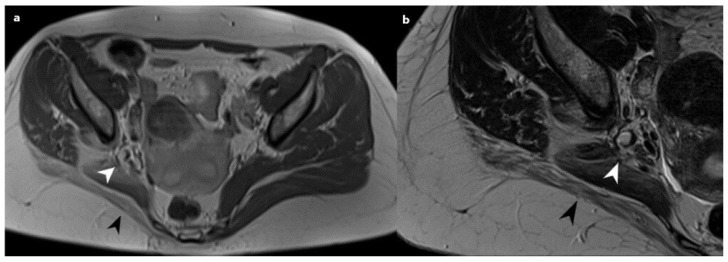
Post-GnRHa treatment; an axial T1 (a) and T2 (b) weighted MRI scan showing a now reduced-sized right sciatic endometriosis nodule (white arrowheads); note gluteal muscle atrophy (black arrowheads).
